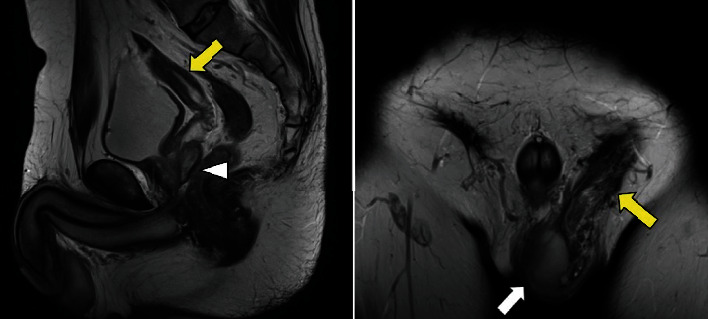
Magnetic resonance imaging shows a left inguinal hernia containing uterine and vaginal structures extending from the left inguinal canal into the scrotum (yellow arrows). There is a normal testis in the left hemiscrotum (white arrow). Müllerian duct derivatives are connected to the prostate on the dorsal side (white arrowhead).
